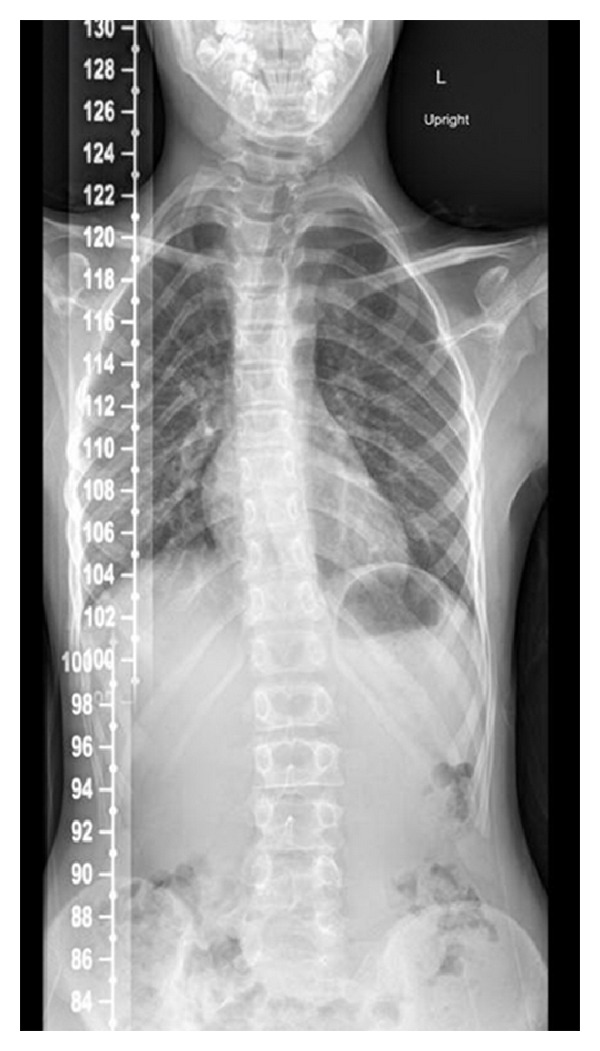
Chest radiograph from patient, showing 13 sets of ribs and “S” shaped scoliosis with 11-degree thoracic dextroconvex and 8-degree lumbar levoconvex scoliosis.
